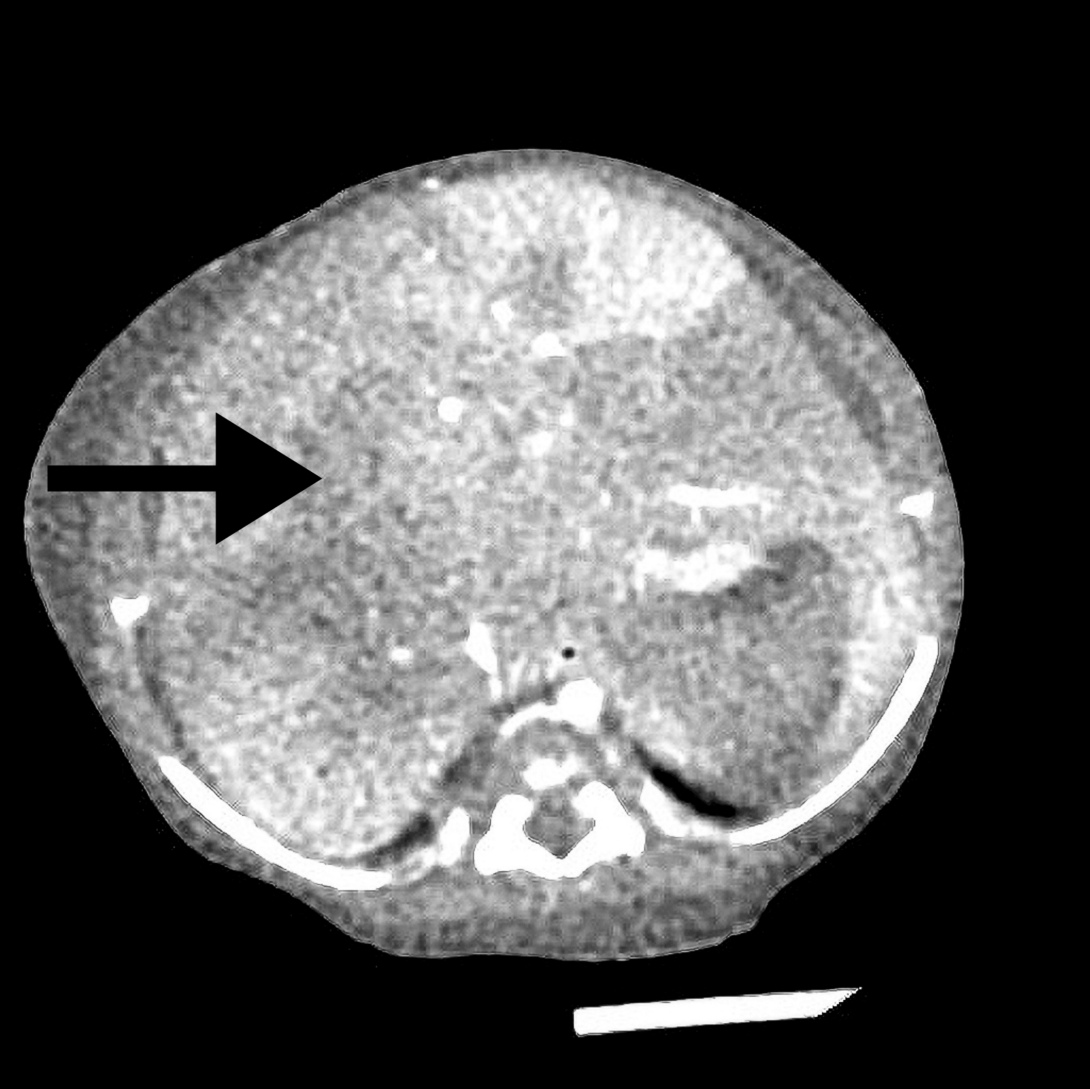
Abdominal CT of the third patient. CT scan showing hepatomegaly. The arrow points to the area of interest.
